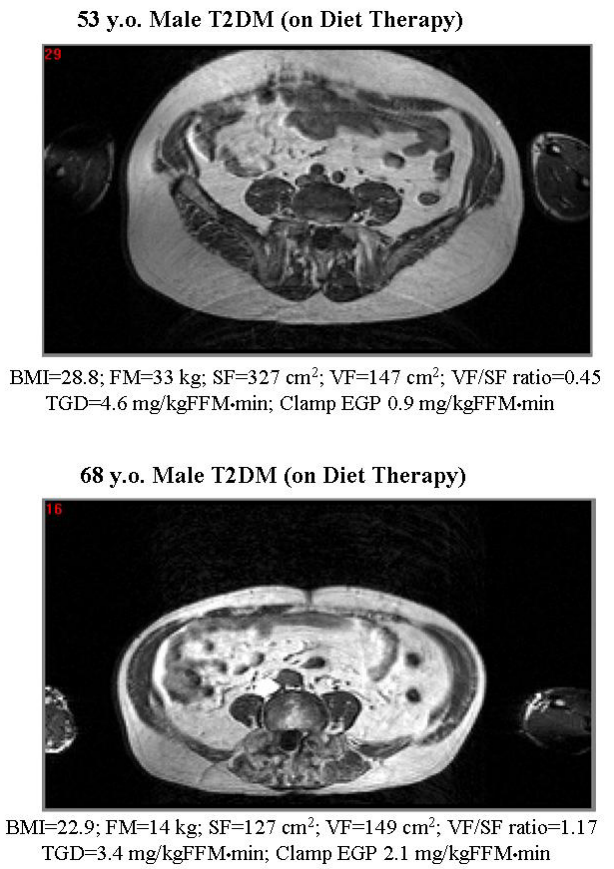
Transverse cross-sectional magnetic resonance image at the L_4–5 _vertebral level in a study subject whose visceral to abdominal subcutaneous fat (VF/SF) ratio was increased (bottom) or decreased (upper).
